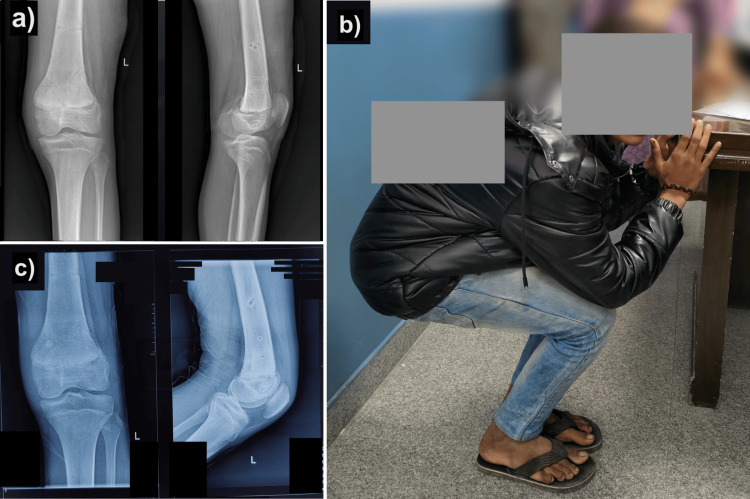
X-ray showing union in an acceptable position at 10 weeks after external-fixator removal (a); the patient could flex knees to 90° at 10 weeks post-operative (b); X-ray showing union at one-year post-operative period (c).
